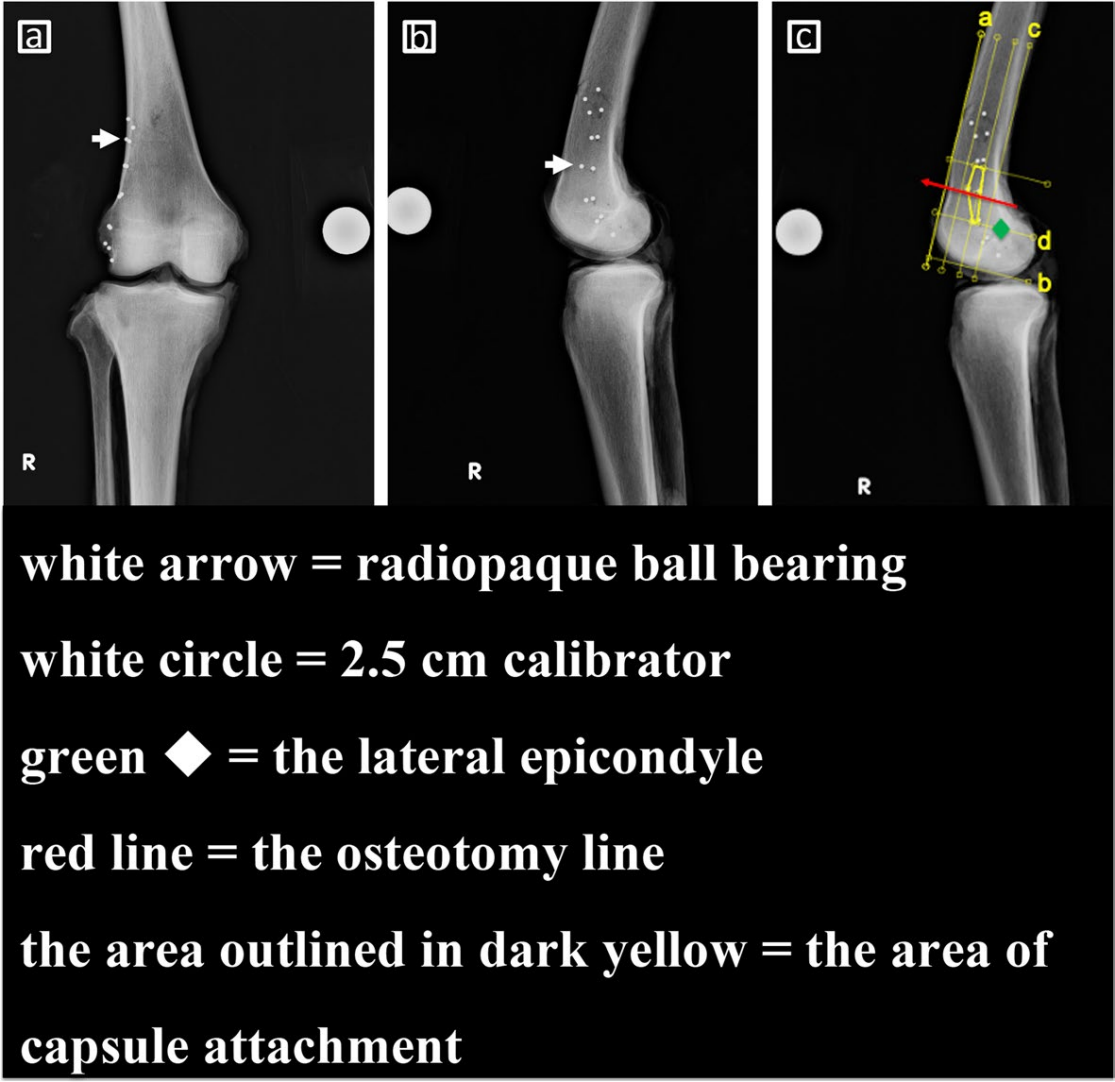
Radiography of the marked capsule attachment of the lateral distal femur. a frontal view, (b) sagittal view, (c) area of capsule attachment around the hinge point of medial closed wedge distal femoral osteotomy (MCWDFO). Line a is parallel to the anterior surface of the femoral shaft. Line b is perpendicular to line a and passes through the most distal part of the lateral distal femoral condyle. Line c is parallel to line a and in contact with the posterior part of the distal femoral shaft. Line d is parallel to line b and passes through the lateral epicondyle. A rectangle centered on the osteotomy line is created by lines a, c, and d. The rectangular area is divided into three regions: front; middle; and rear. The area of capsule attachment in the middle and rear regions is calculated using a digital planning tool
